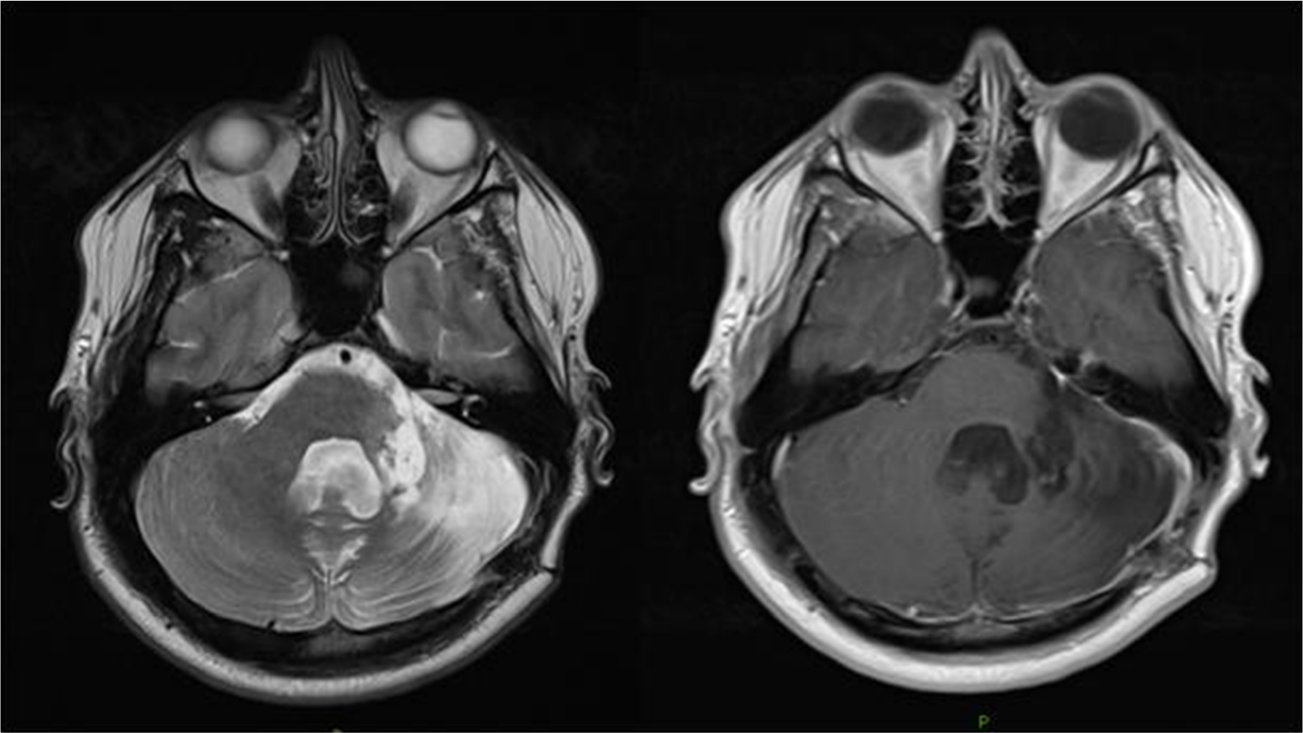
Post-operative axial T2 (left) and T1 MRI (right) brain post-contrast at 9 months post-resection. Evidence of associated gliosis with some haemosiderin deposit in the left cerebellar hemisphere. No pathological enhancement is seen in the area of the resection cavity to indicate tumour recurrence
